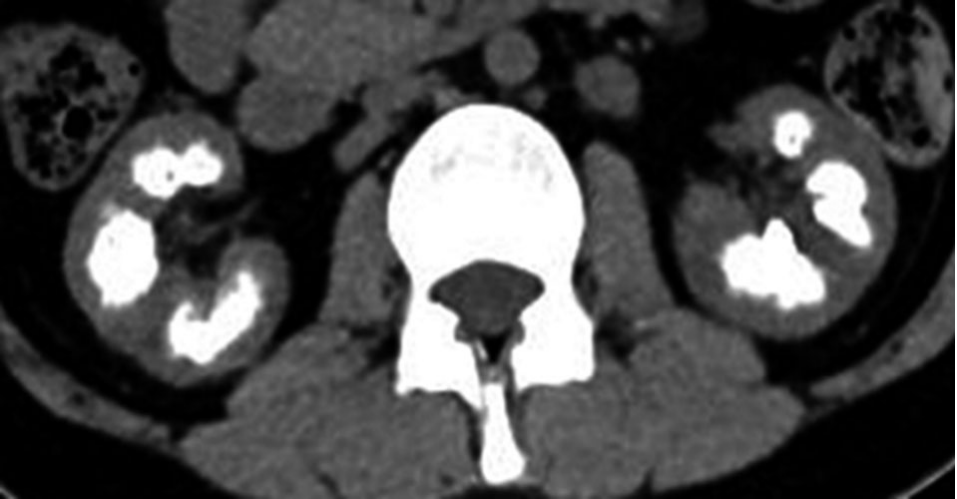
Nephrocalcinosis of native kidneys. Abdominal CT revealed severe calcification of the bilateral kidneys in October 20XX. Nephrocalcinosis was not improved although the urine calcium levels maintained a normal range thereafter. In this case, tumoral calcinosis was found only in the kidney.
